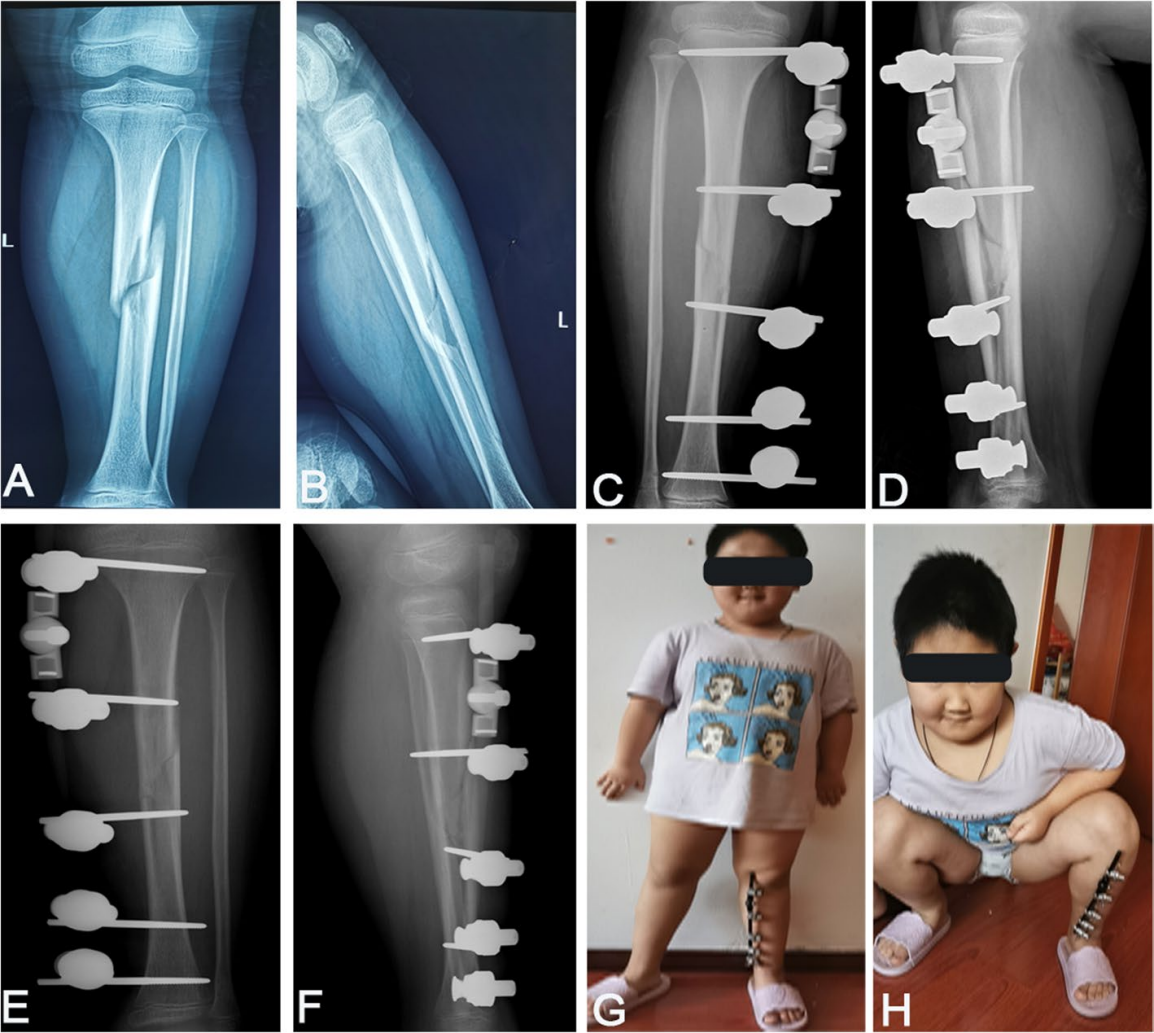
Representative cases with tibia shaft fractures at preoperation and postoperation. A, B X-ray images of tibia shaft fractures of an 8-year-old boy. C, D X-ray images at postoperative immediate showed good reduction. E, F X-ray images at postoperative 1 month. G, H At 5 weeks postoperatively, the patient walked without crutches and recovered well
